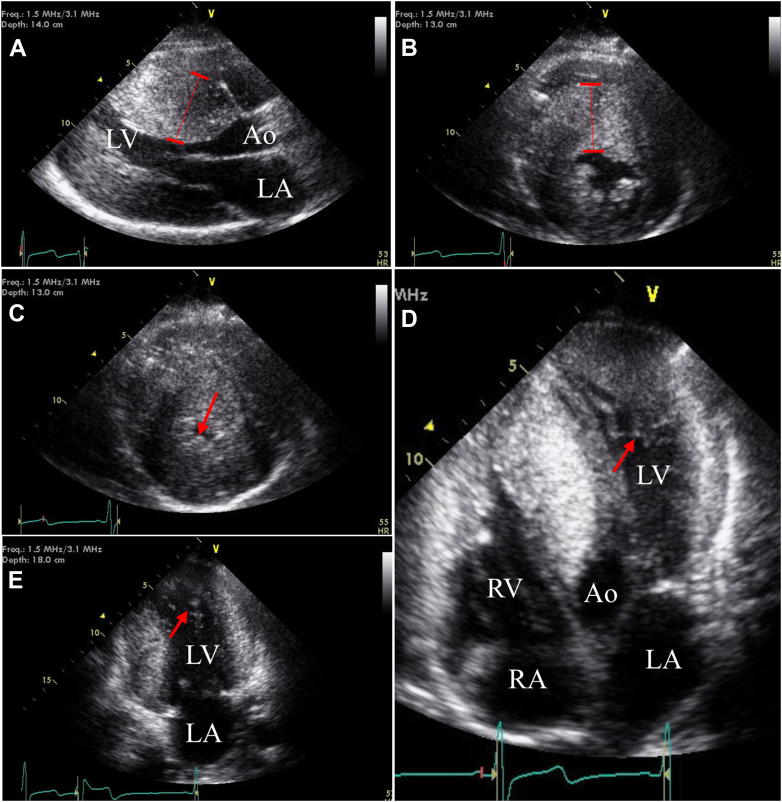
Hypertrophic Cardiomyopathy With Marked Asymmetric Septal Hypertrophy The patient's echocardiographic images at age 19. Parasternal long- (A) and short-axis (B) views of the LV in diastole with marked septal hypertrophy, measuring 3.5 cm. A short-axis view of the LV in systole with cavity obliteration (C). Apical 4- (D) and 2-chamber (E) views. On current review of imaging, evidence of trabeculation without recesses is present (red arrows in C to E). Ao = aorta; LA = left atrium; LV = left ventricle; RA = left atrium; RV = right ventricle.
